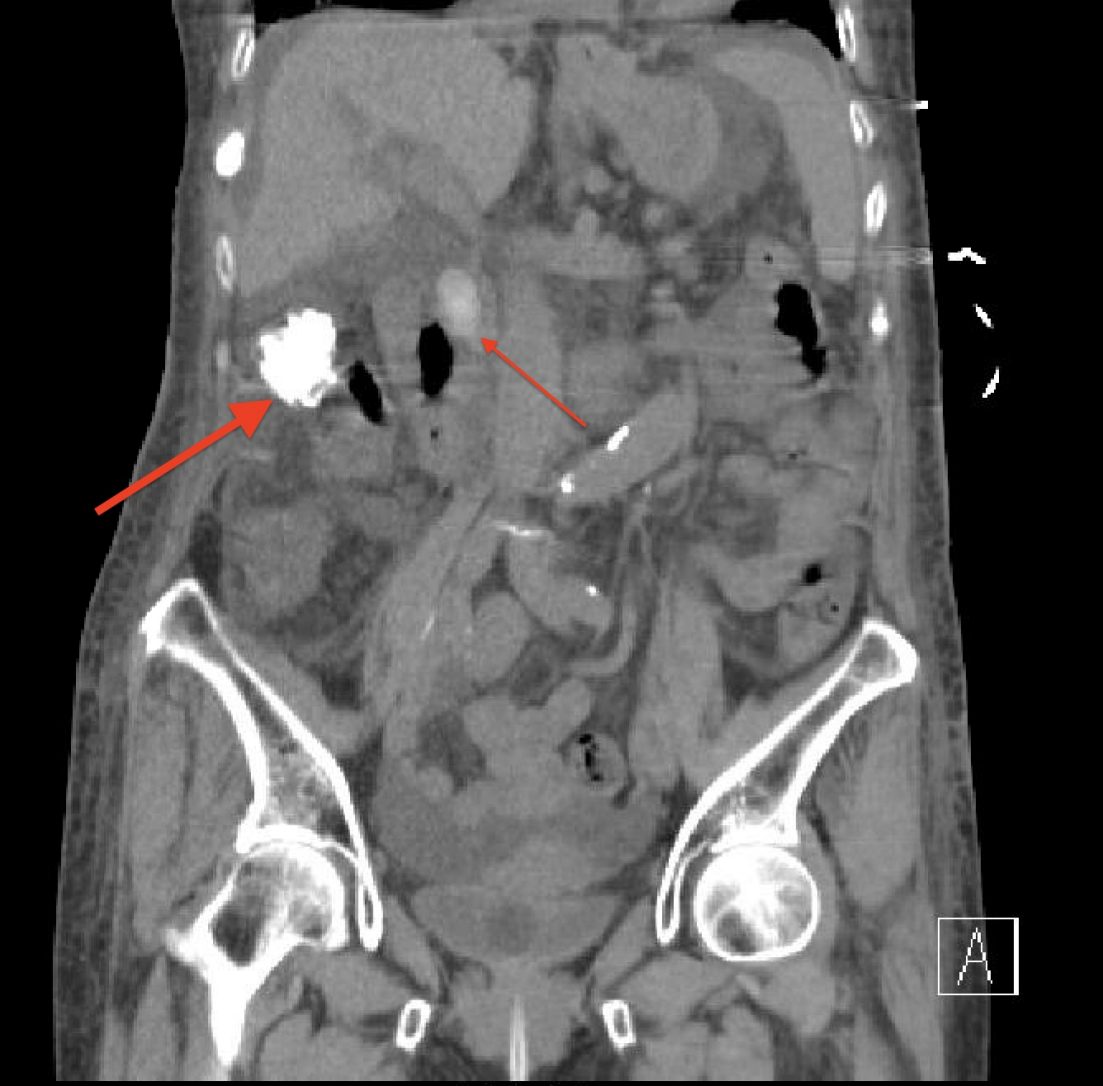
Computed tomography coronal image showing proximal/mid-common bile duct stone (thin arrow) Additional layering stones (thick arrow) in the gallbladder are also visualized. Intra and extra-hepatic ductal dilatation is seen.
